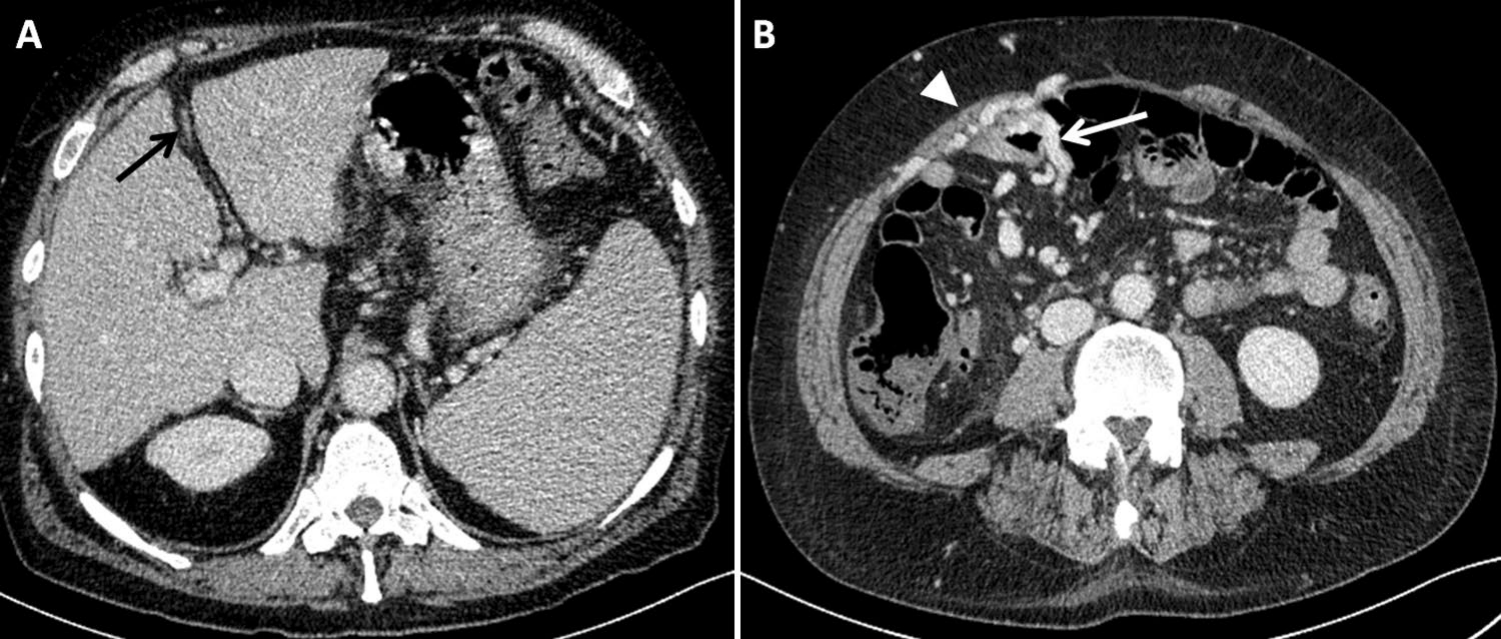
A–B Venous phase CT scan at the level of the portal vein bifurcation A shows cirrhotic liver with the fibrotic remnant of the umbilical vein (black arrow) in the falciform ligament after thrombosis due to cholecystitis; splenomegaly due to portal hypertension is clearly visible. Venous phase CT scan of the paraumbilical region B depicts engorged branches of the superior mesenteric vein in connection with submucosal varices protruding into the lumen of the proximal ileum (white arrow); enlarged right epigastric veins (white arrowhead) are also demonstrated in the anterior abdominal wall
